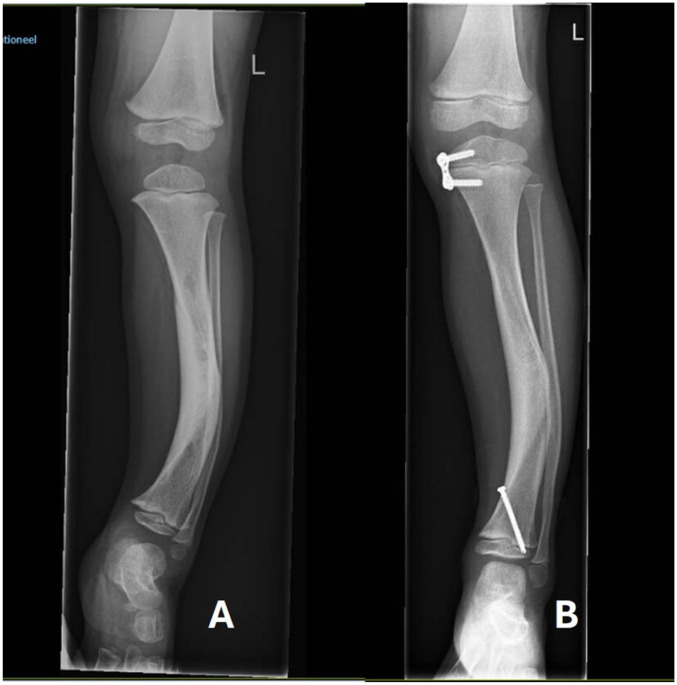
Radiographs of anterolateral bowing of the tibia in a patient with neurofibromatosis 1. Preoperative (a) and after 2-year follow-up (b). Distally, the choice of implant used was based on anatomical landmarks; the tension band plate was not feasible on the lateral side of the tibia due to the presence of the fibula.
